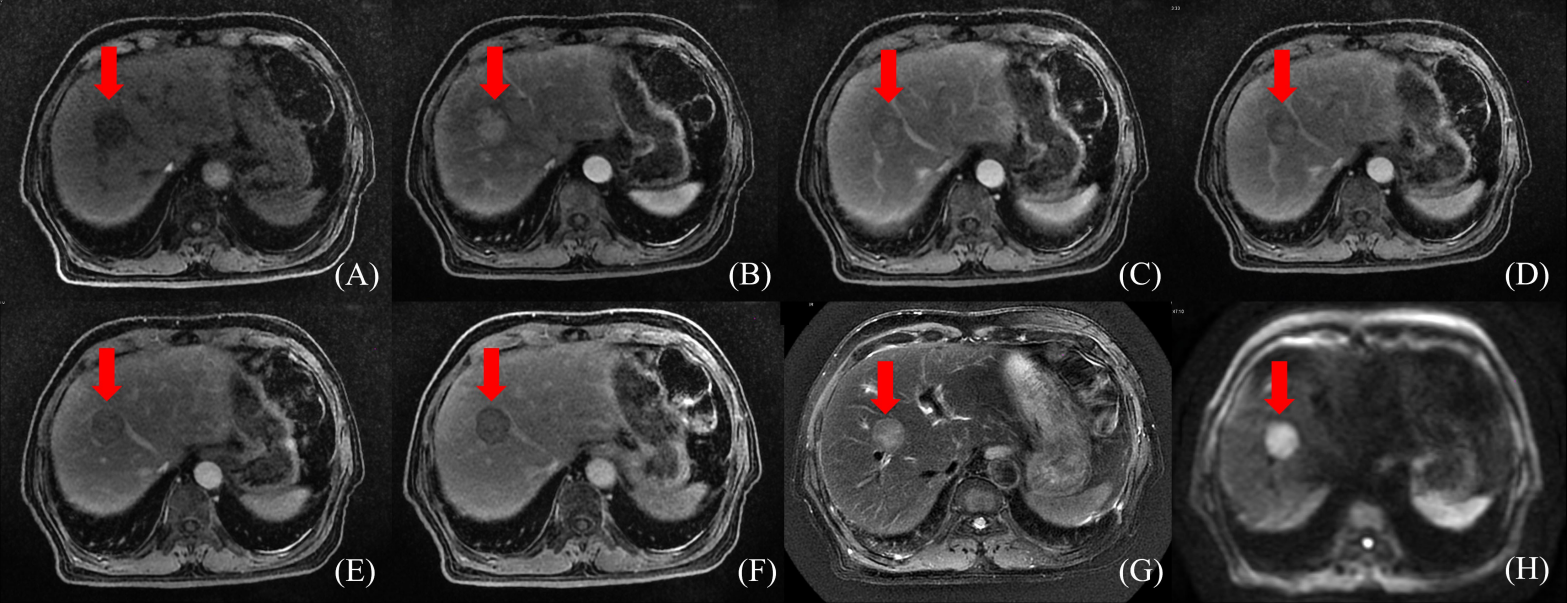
HCC MR border imaging. (A) T_1_-weighted Image without contrast (B–F) T_1_-weighted Image after contrast agent 15s,45s,75s,150s,10min (G) T_2_ –weighted Image with fat suppression (H) Diffusion Weighted Imaging (DWI).
